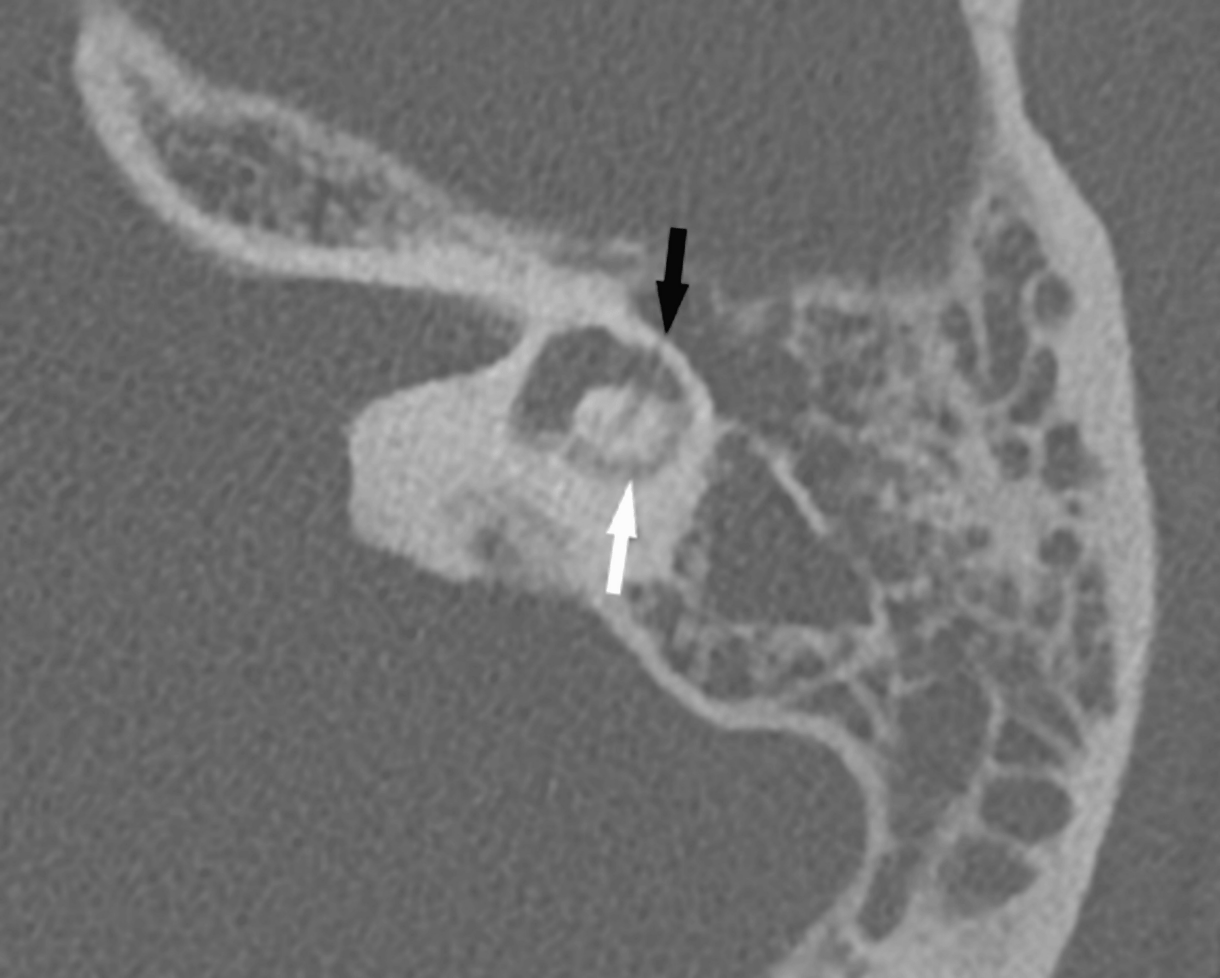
High-resolution axial CT scan of the left temporal bone; fracture involving the lateral semi-circular canal associated with ossification Black arrow: fracture; white arrow: mild ossification of the lateral canal
